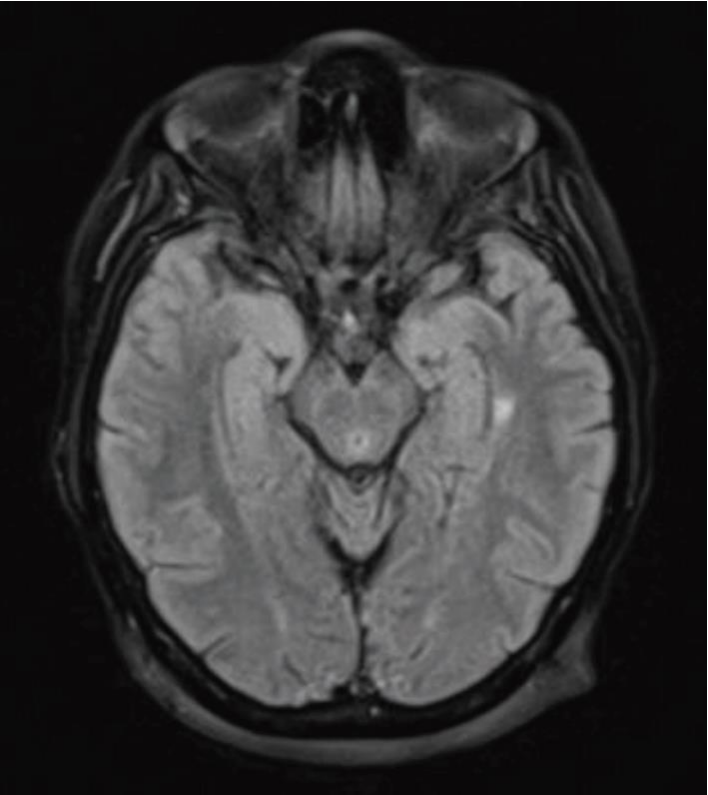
Axial T2 FLAIR MRI, series 7, image 13/32. Juxtacortical left parietal lobe lesion.
